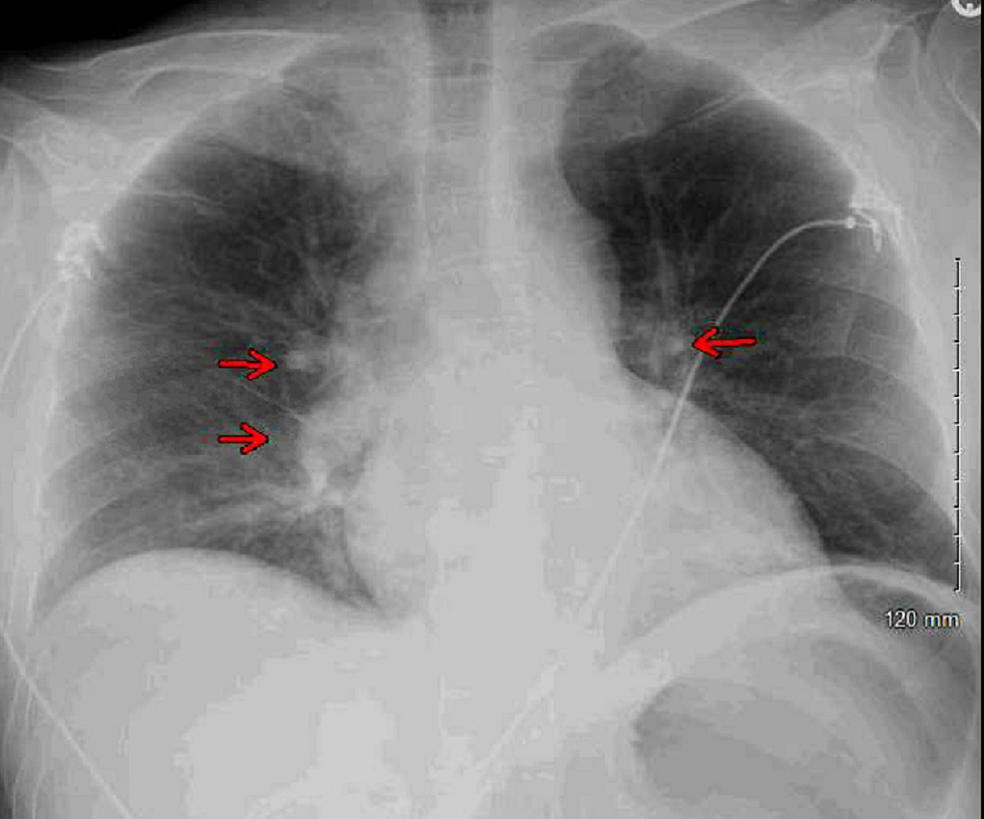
Chest X-ray revealed bilateral perihilar patchy and hazy airspace opacities (red arrows).
